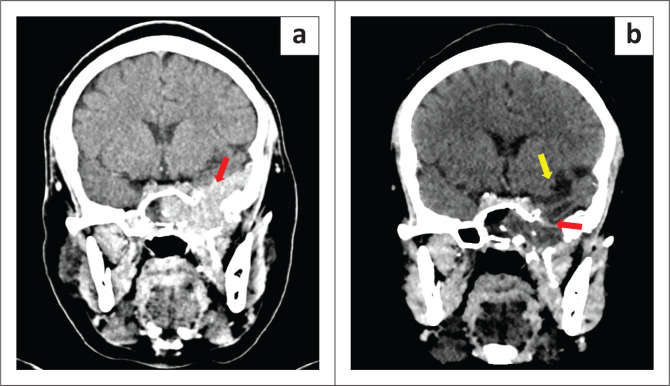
(a, b) Post-therapeutic post-contrast coronal CT head at 5 months (a) and 9 months (b) after diagnosis. Post-chemotherapy image (a) shows a decrease in the volume of the base of skull plasmacytoma (red arrow). Post-radiation image (b) shows a radiological response with no residual enhancing tumour (red arrow). There is post-therapeutic left temporal lobe volume loss (yellow arrow).
